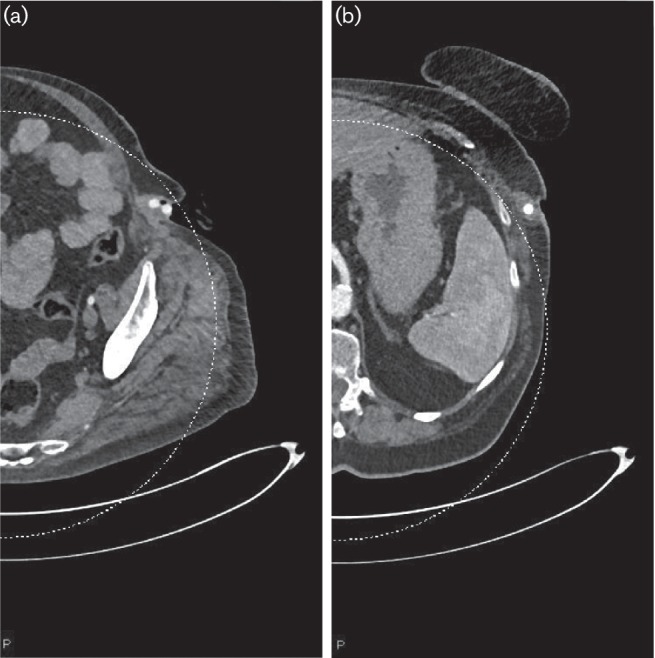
Axial images of a CT angiogram of the whole aorta and lower limbs showing (a) the graft exposed in the left groin and (b) an area of fluid or soft tissue attenuation surrounding the graft in the left axilla.
